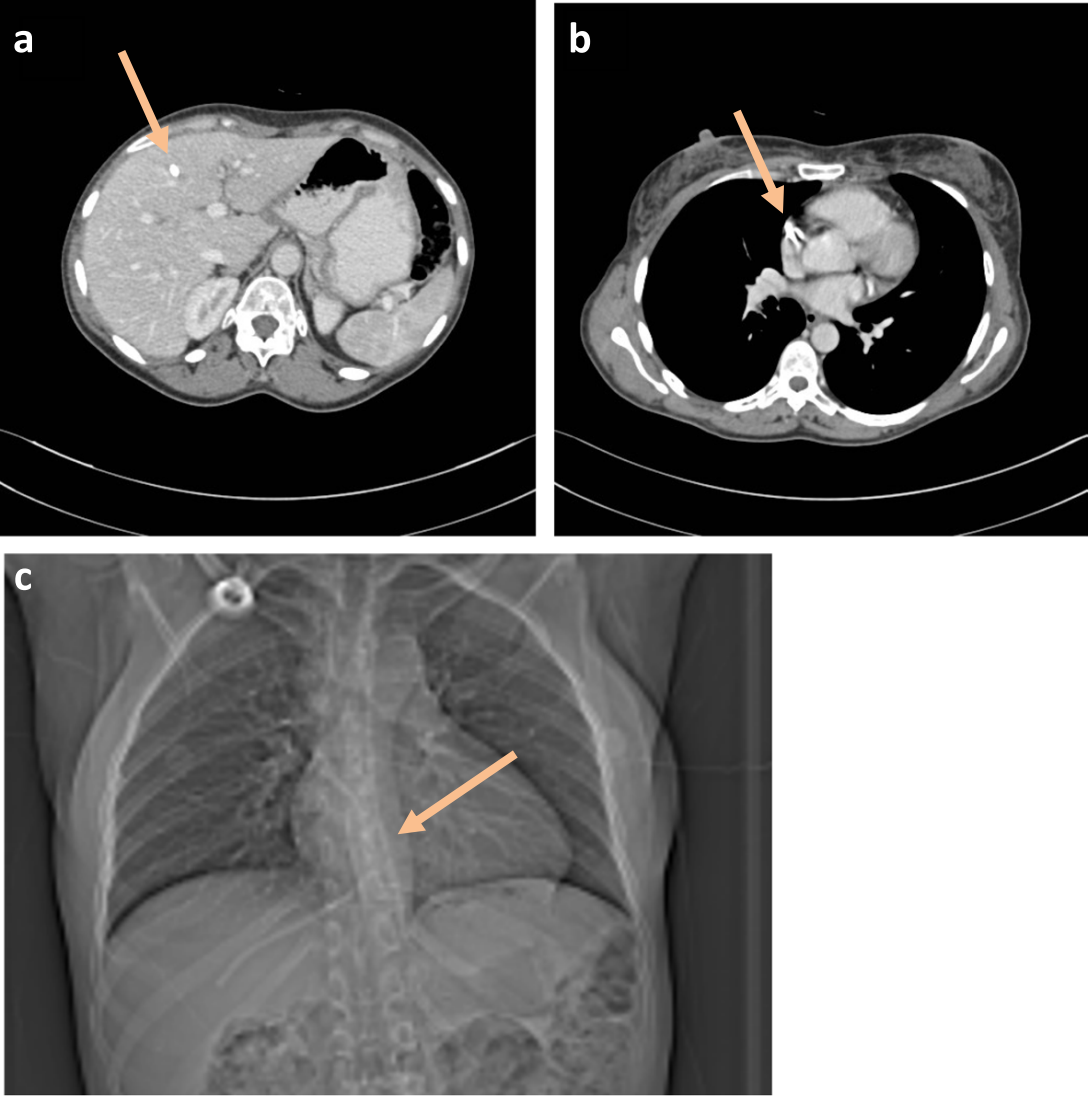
Contrast enhanced axial images of the chest and abdomen demonstrating the distal tip of the catheter fragment in the middle hepatic vein (a, orange arrow) and proximal tip in the right atrial appendage (b, orange arrow). Scanogram of the CT chest and abdomen demonstrating the catheter fragment (c, orange arrow)
